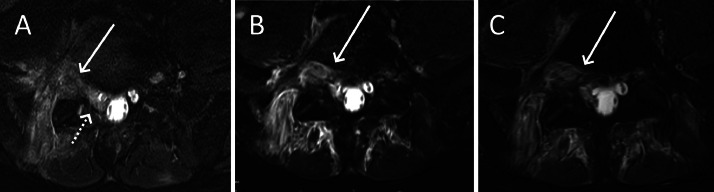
Axial T2 Dixon (water)-weighted image at the L5-S1 level showing the residual thickening around the right nerve root (solid arrow) during the 3rd MRI one month after the start of treatment with persistent intrathecal disease (dotted arrow) (A), the 4th MRI six weeks after the start of treatment (B) and the 5th MRI two months and a half after the start of treatment (C).
